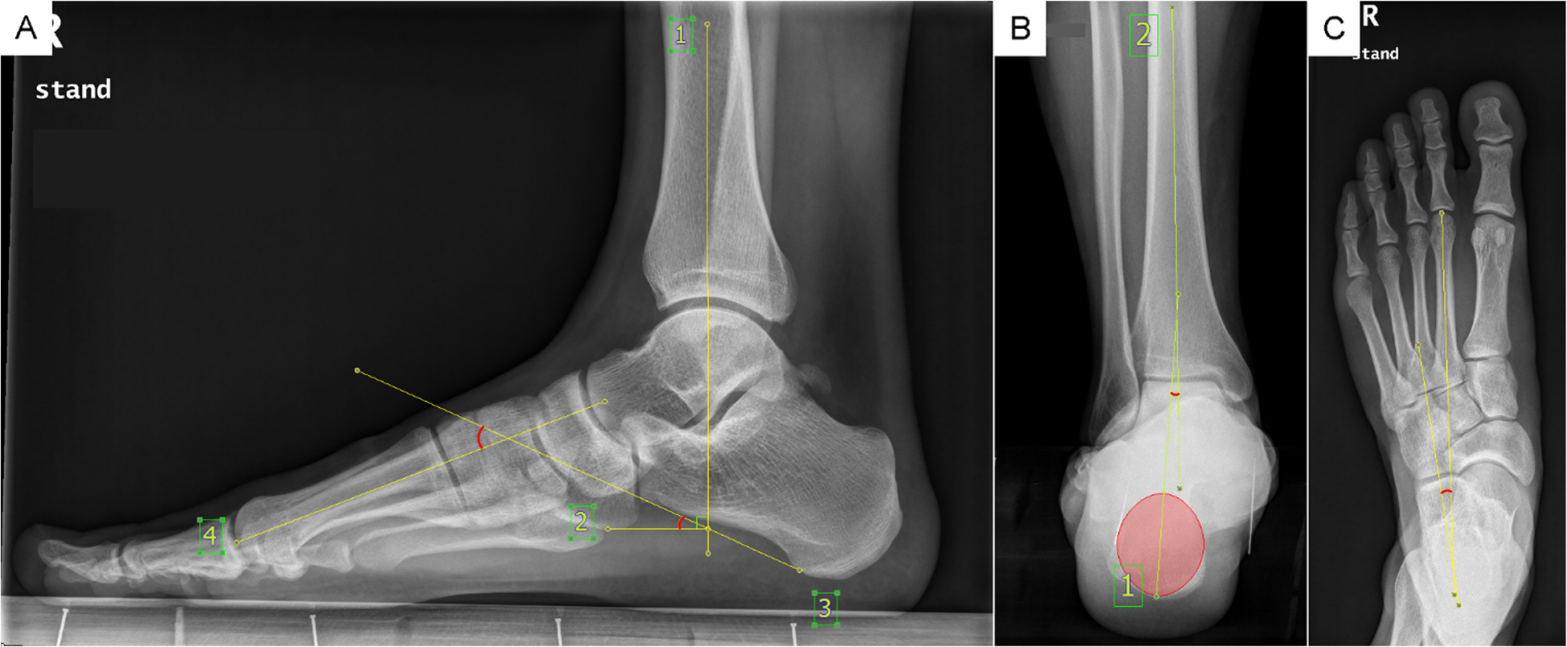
Radiographic measurements from the lateral view (A), posterior view (B), and standing A/P (C)
